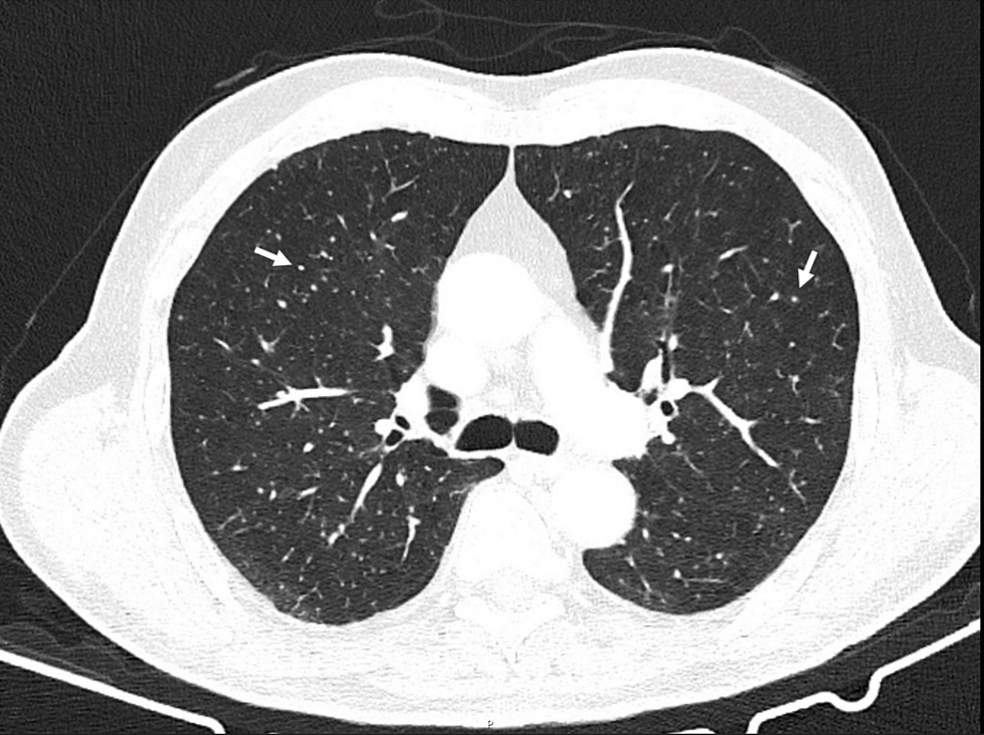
Thoracic CT scan showing pulmonary micronodulation (arrows) in a miliary pattern
